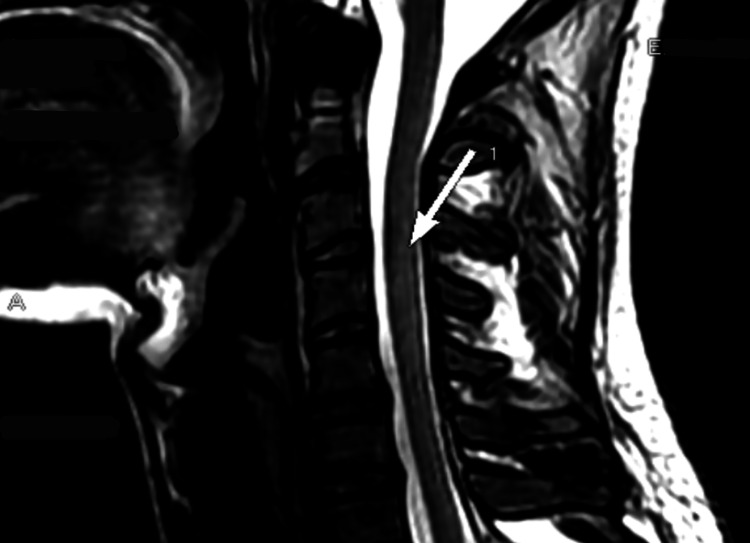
T2-weighted sagittal MRI of the cervical spine revealing hyperintense lesions in the dorsal columns of the spinal cord.
